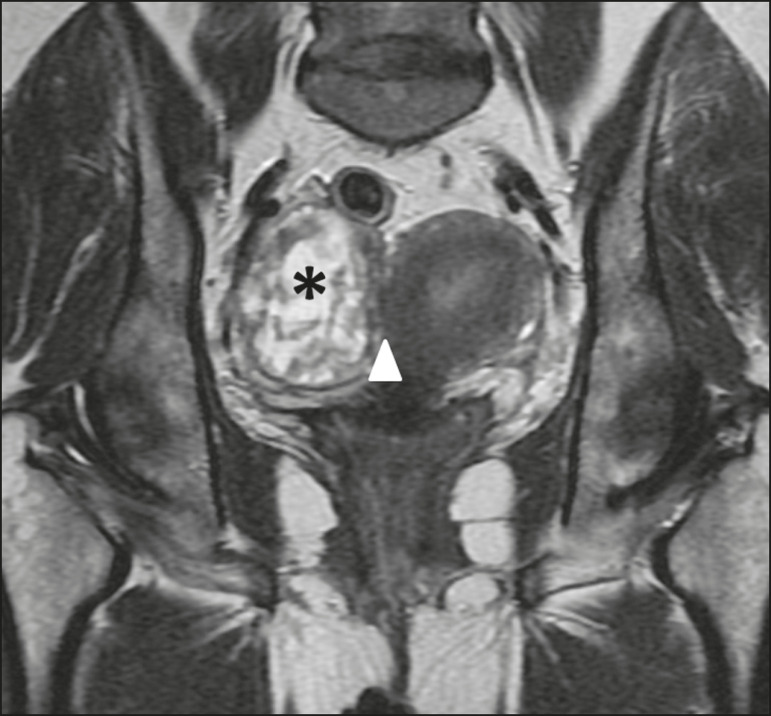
Leiomyoma of the broad ligament with cystic degeneration in a 47-yearold woman. Axial T2-weighted imaging showing a tumor with cystic areas as portions of high T2 signal (asterisk), clearly demarked from the uterus (triangle).
